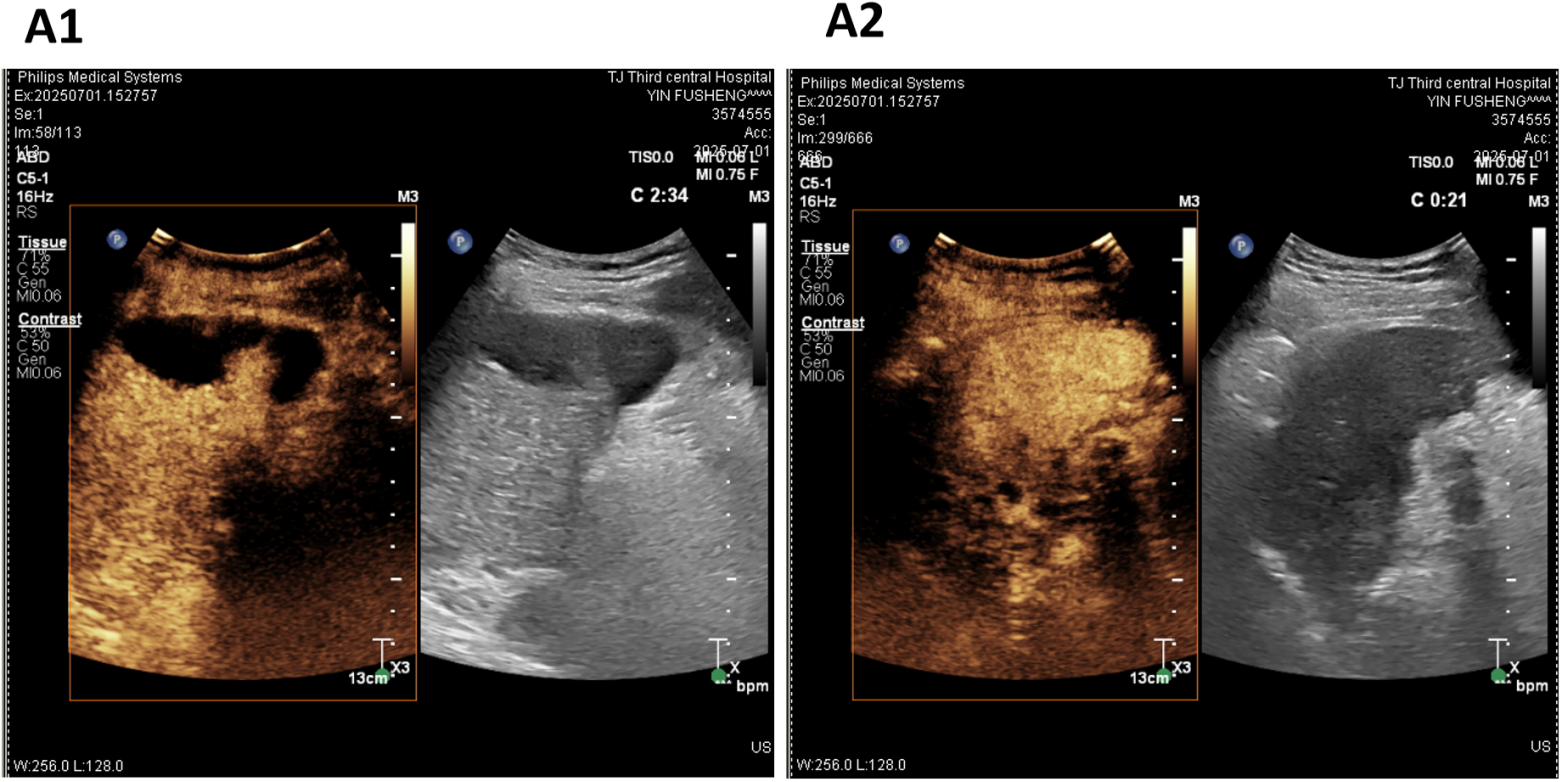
Contrast-enhanced ultrasound (CEUS) images after radiological complete response. (A1, A2) CEUS (July 1, 2025): 1.5 ml of SonoVue was administered via bolus injection through the left median cubital vein. Starting from 12 seconds into the hepatic arterial phase, no contrast agent enhancement was observed in multiple intrahepatic lesions during the arterial phase, portal venous phase, or delayed phase, with clear boundaries. The embolus in the right portal vein showed no enhancement in the arterial phase, contrast agent inflow during the portal venous phase, and hypoenhancement in the delayed phase. No additional washout lesions were detected throughout the liver during the delayed phase scanning. CEUS, Contrast-Enhanced Ultrasound.
